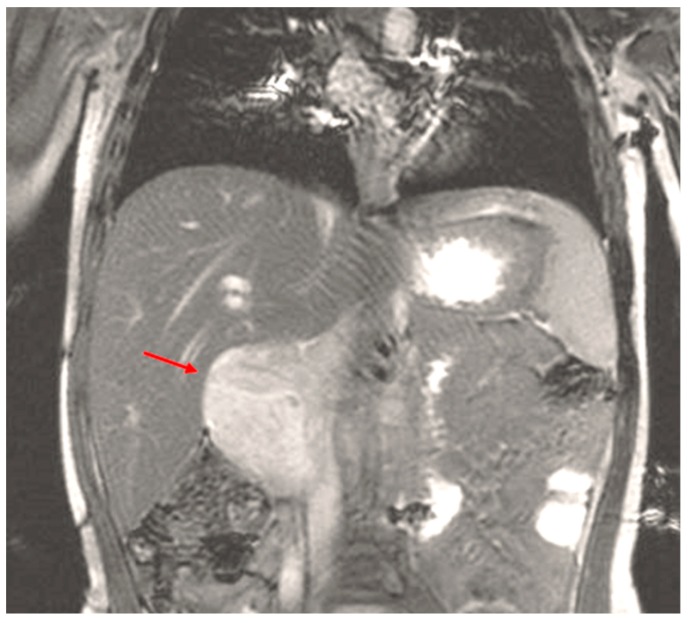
MRI (Coronal View) of patient with hepatobiliary embryonal rhabdomyosarcoma (RMS) (red arrow).
